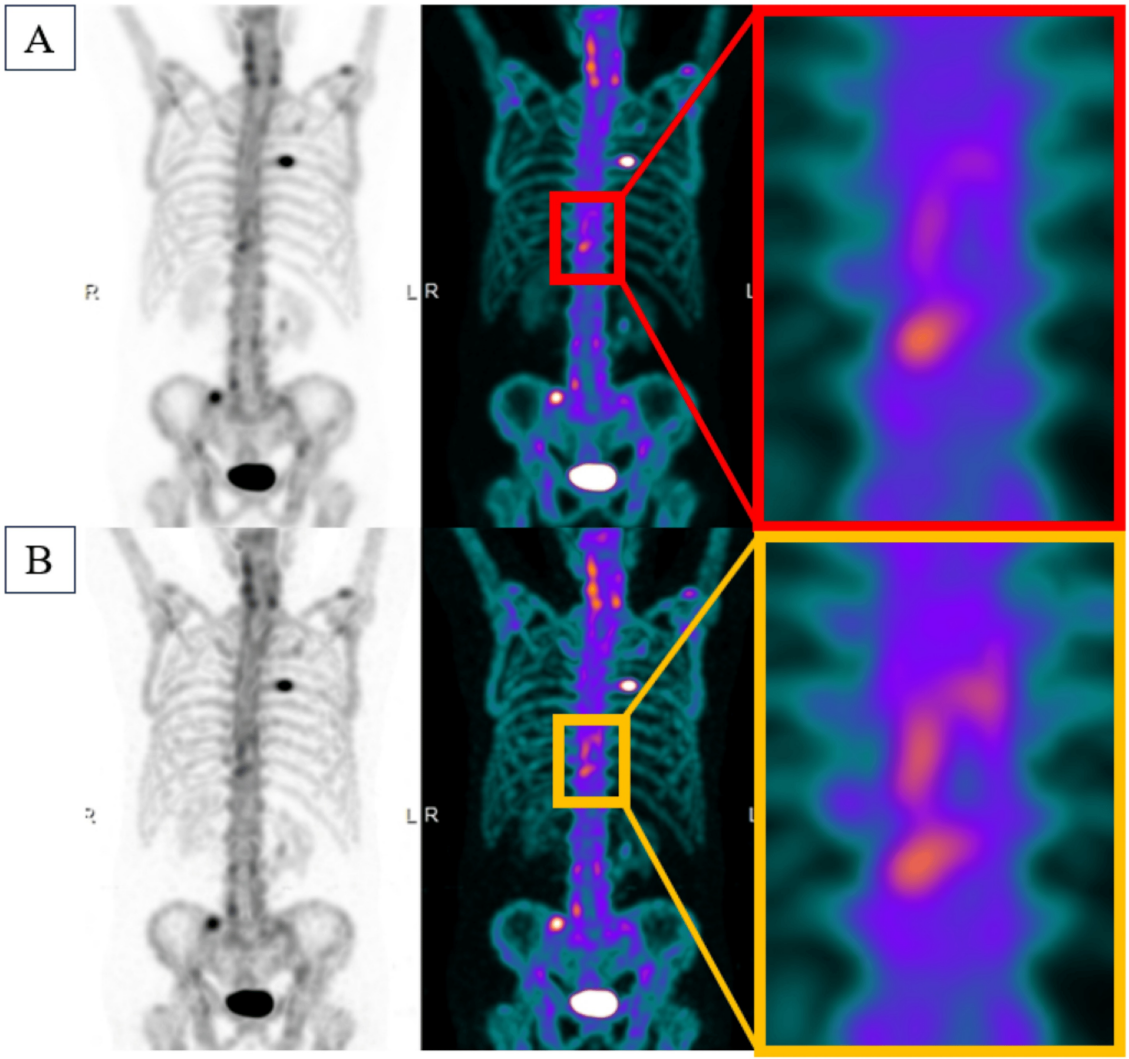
Bone scintigraphy images These are the images processed using the conventional method (A) and the images processed using xSPECT (B). The xSPECT-processed images exhibit higher contrast than those processed using the conventional method. xSPECT, extended single-photon emission computed tomography (SPECT)
